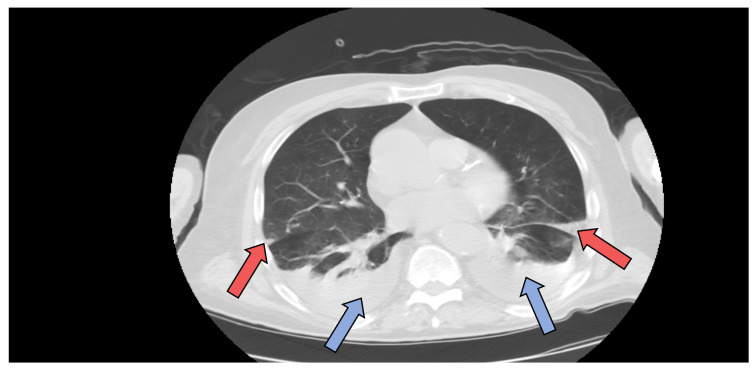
Chest computer tomography (CT) axial view showing bilateral pulmonary infarction (red arrows) and bilateral pleural effusion (blue arrows).
